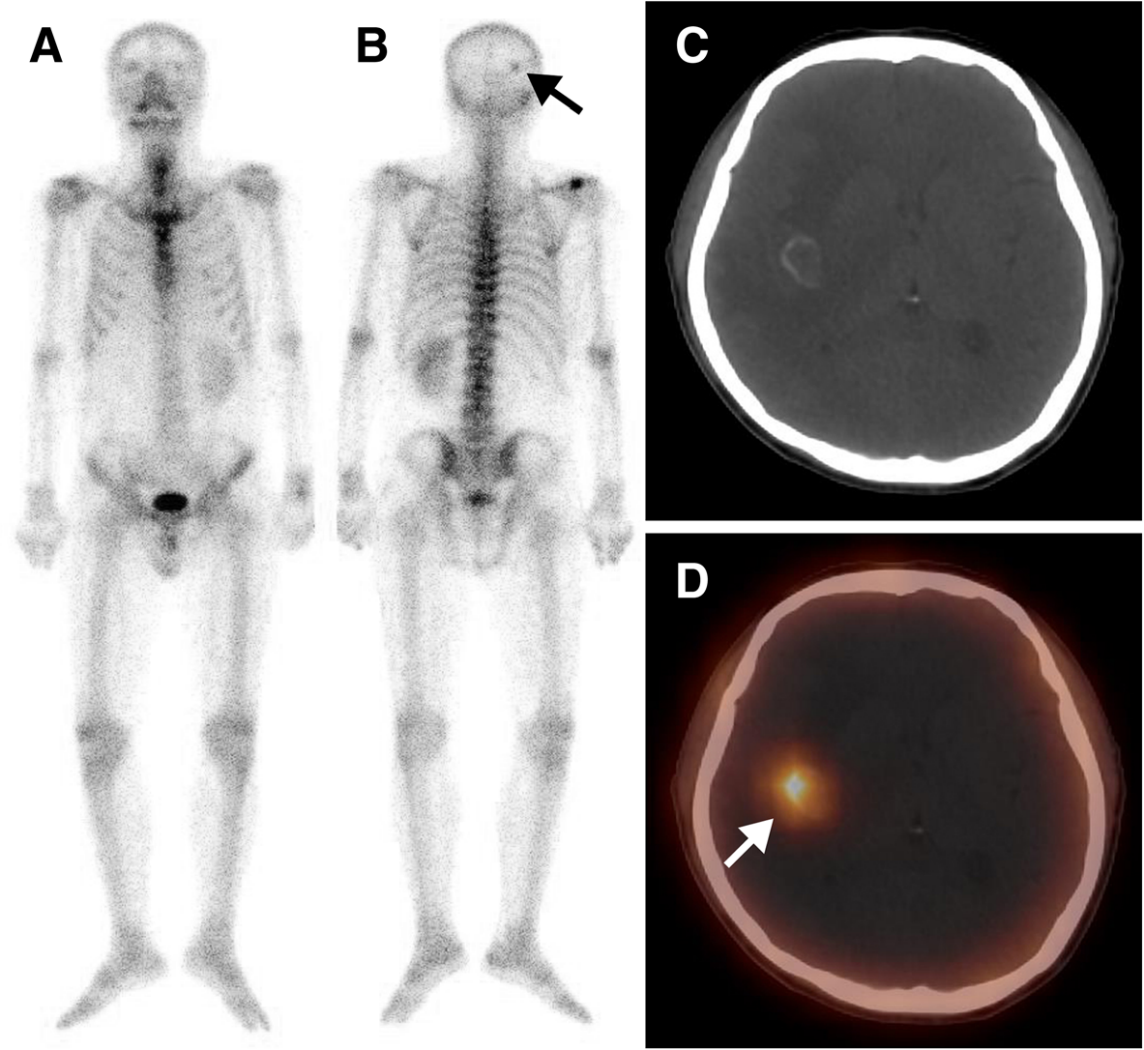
A 49-year-old man with lung cancer (adenocarcinoma) after surgery and chemotherapy for 1 year, who was referred for suspected metastasis. The whole-body scan (a, anterior; b, posterior) revealed a single focus of high ^99m^Tc-MDP activity in the right occipital bone (black arrow). Axial CT (c), and SPECT/ CT images (d) showed elevated ^99m^Tc-MDP activity in a brain metastasis at the grey-white matter junction in the right parietal lobe (white arrow)
